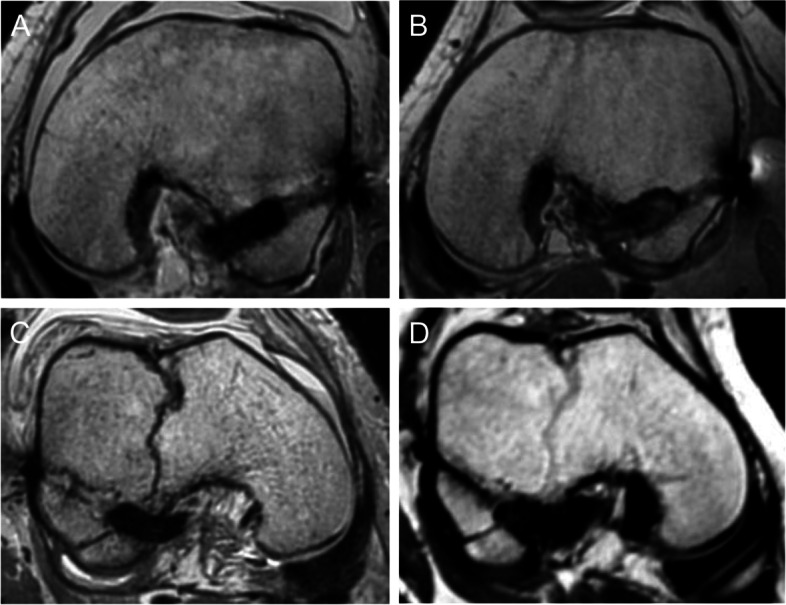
Magnetic resonance images. A An image of the left knee of a 43-year-old female one week after surgery shows that the hamstring graft was completely inserted in the socket. B An image of the left knee of a 43-year-old female one year after surgery (same case of A) shows that there is a gap between the top of the femoral socket and the graft. C An image of the right knee of a 14-year-old female one week after surgery shows that the hamstring graft is not completely inserted. D An image of the right knee of a 14-year-old female one year after surgery (same case of C) shows that there is no gap between the top of the femoral socket and the graft
